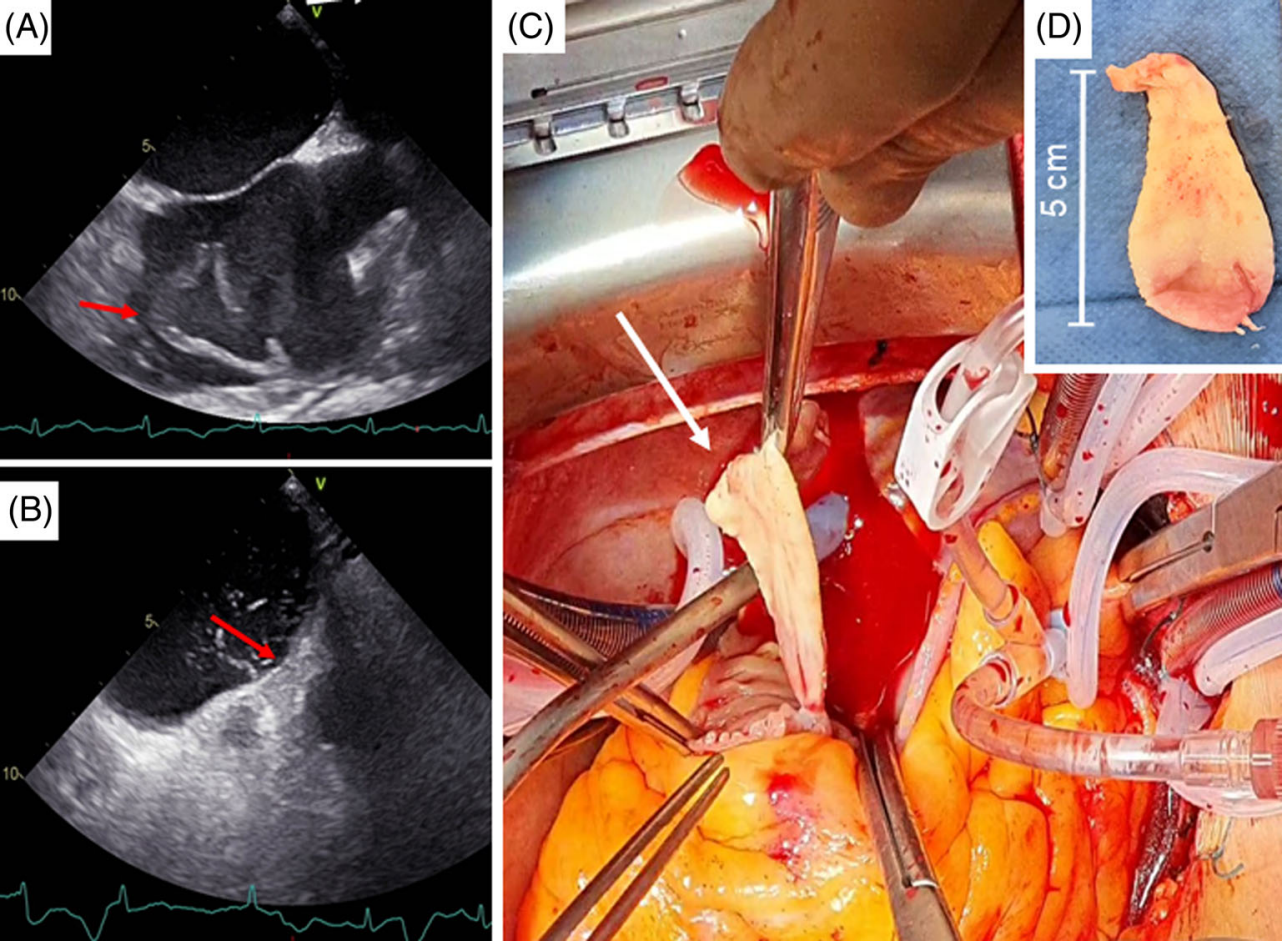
Clinical documentation of findings. (A) Transesophageal echocardiography (TEE) displaying metastasis of the right atrium (red arrow). (B) TEE documented PFO (red arrow). (C) Intraoperative photography of the metastasis in the right atrium (white arrow). (D) Photography of resected metastasis
